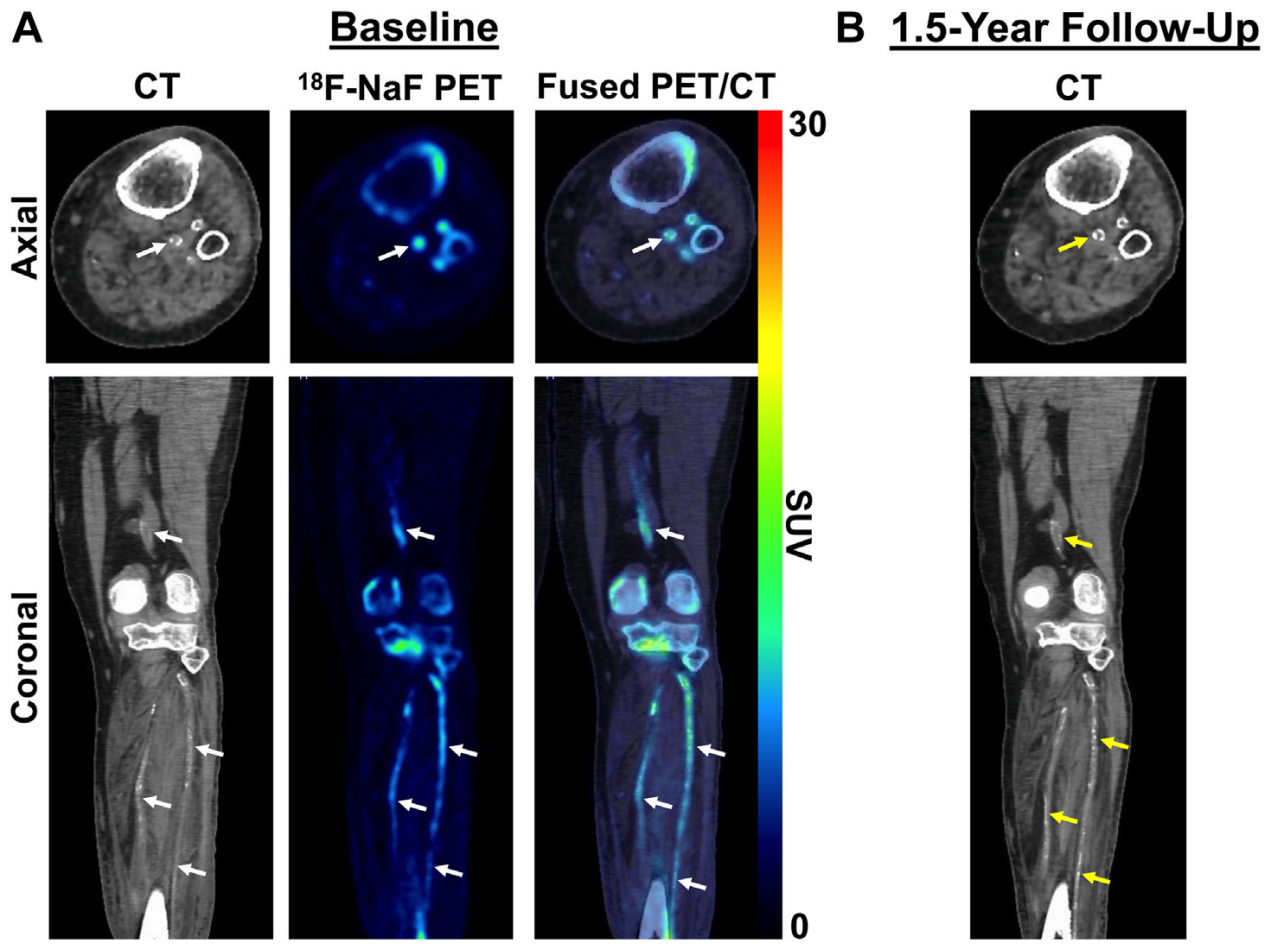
Fluorine-18 (^18^F)-sodium fluoride (NaF) positron emission tomography (PET)/computed tomography (CT) imaging of multivessel calcific disease progression. A, Baseline PET/CT imaging at study enrollment revealing various levels of CT-detectable calcium deposition in the popliteal, posterior tibial, anterior tibial, and peroneal arteries (*white arrows*) with active microcalcification occurring in multiple vessels, as shown by ^18^F-NaF PET imaging (*white arrows*). B, Follow-up CT imaging 1.5 years later demonstrating new calcium or progression of existing calcium within vascular regions (*yellow arrows*) that were previously identified as having elevated levels of ^18^F-NaF uptake (ie, active disease). *SUV,* Standardized uptake value.
